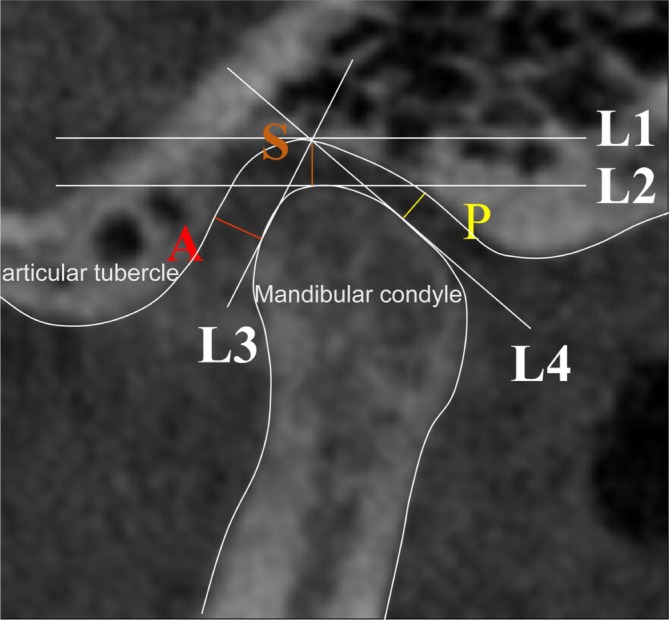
CBCT measurement based on Kamelchuk's method. L1: Horizontal line parallel to the Frankfort Horizontal (FH) plane, tangent to the roof of the glenoid fossa. L2: Horizontal line parallel to the FH plane, tangent to the superior edge of the condyle. L3: Tangent line to the anterior surface of the condyle. L4: Tangent line to the posterior surface of the condyle. A, Anterior joint space; P, Posterior joint space; S, Superior joint space.
